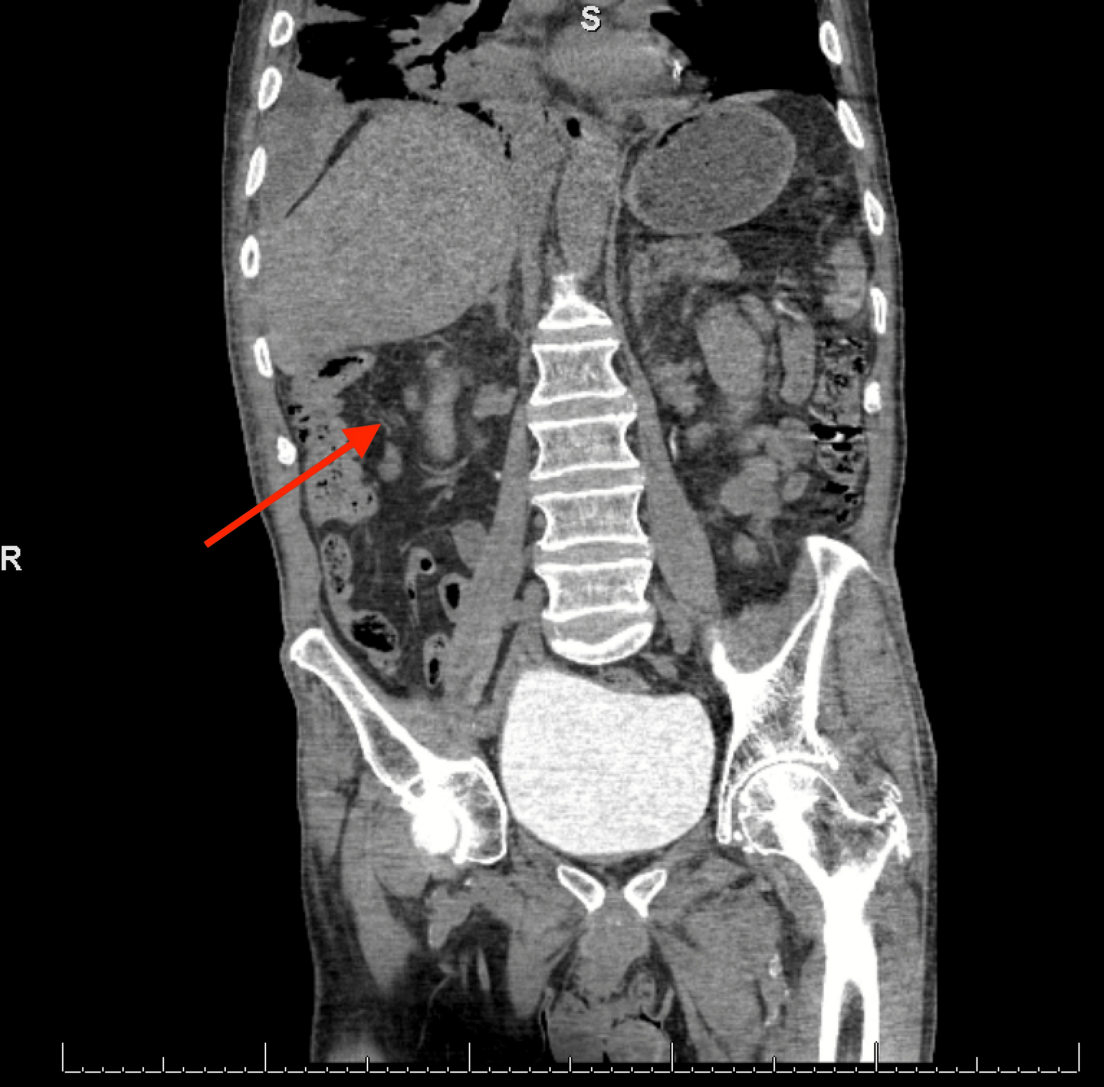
CT of the abdomen and pelvis showing a fluid-filled structure within the area of the gallbladder fossa
